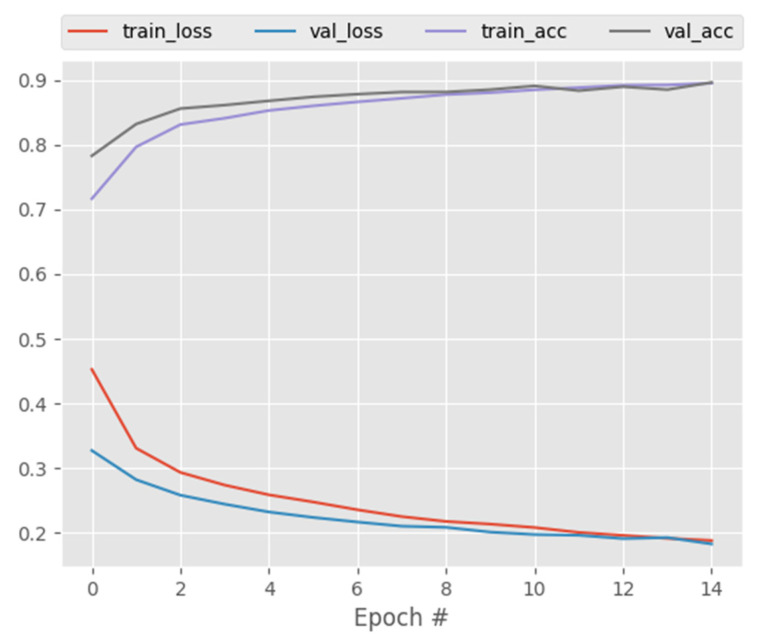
DenseNet121 training and validation performance for X-ray images.
